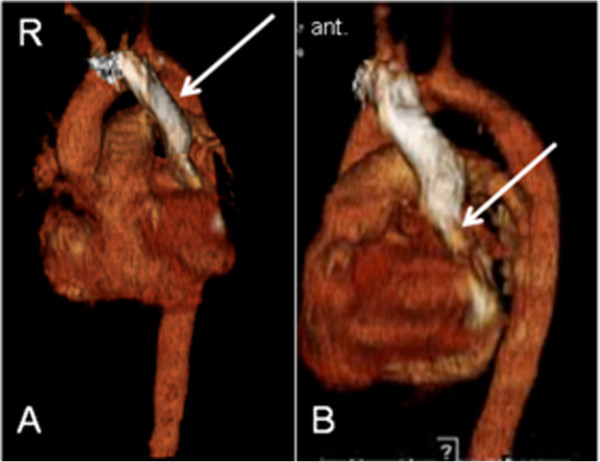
Persistent left superior vena cava in CT in 3D-reconstruction. A: 3D-reconstruction view from anterior left-sided showing the heart anatomy and the relation to the persistent left superior vena cava. B: Lateral view from the left side showing the entrance to the coronary sinus.
